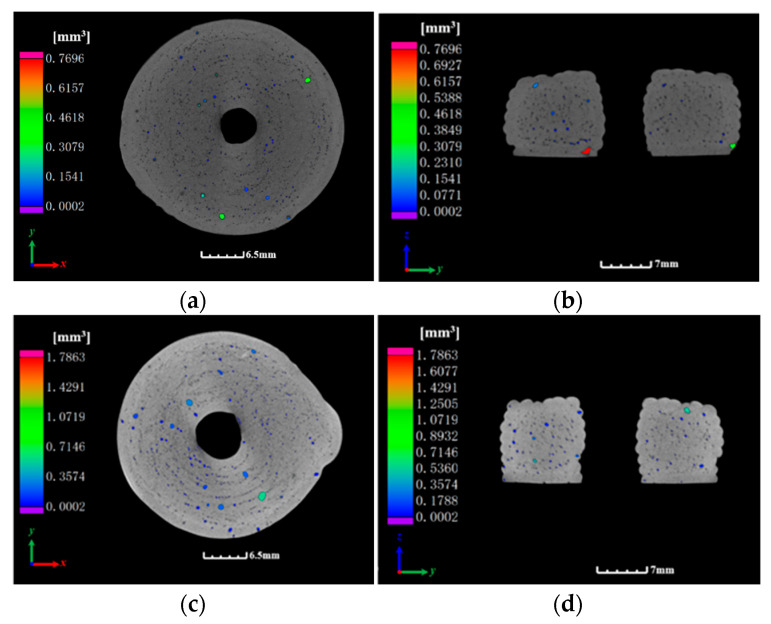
CT test results of substitute propellant grains: (a) radial cross-section of propellant grains formed by plunger extrusion; (b) radial cross-section of propellant grains formed by plunger extrusion; (c) radial cross-section of propellant grains formed by screw extrusion; (d) radial cross-section of propellant grains formed by screw extrusion.
